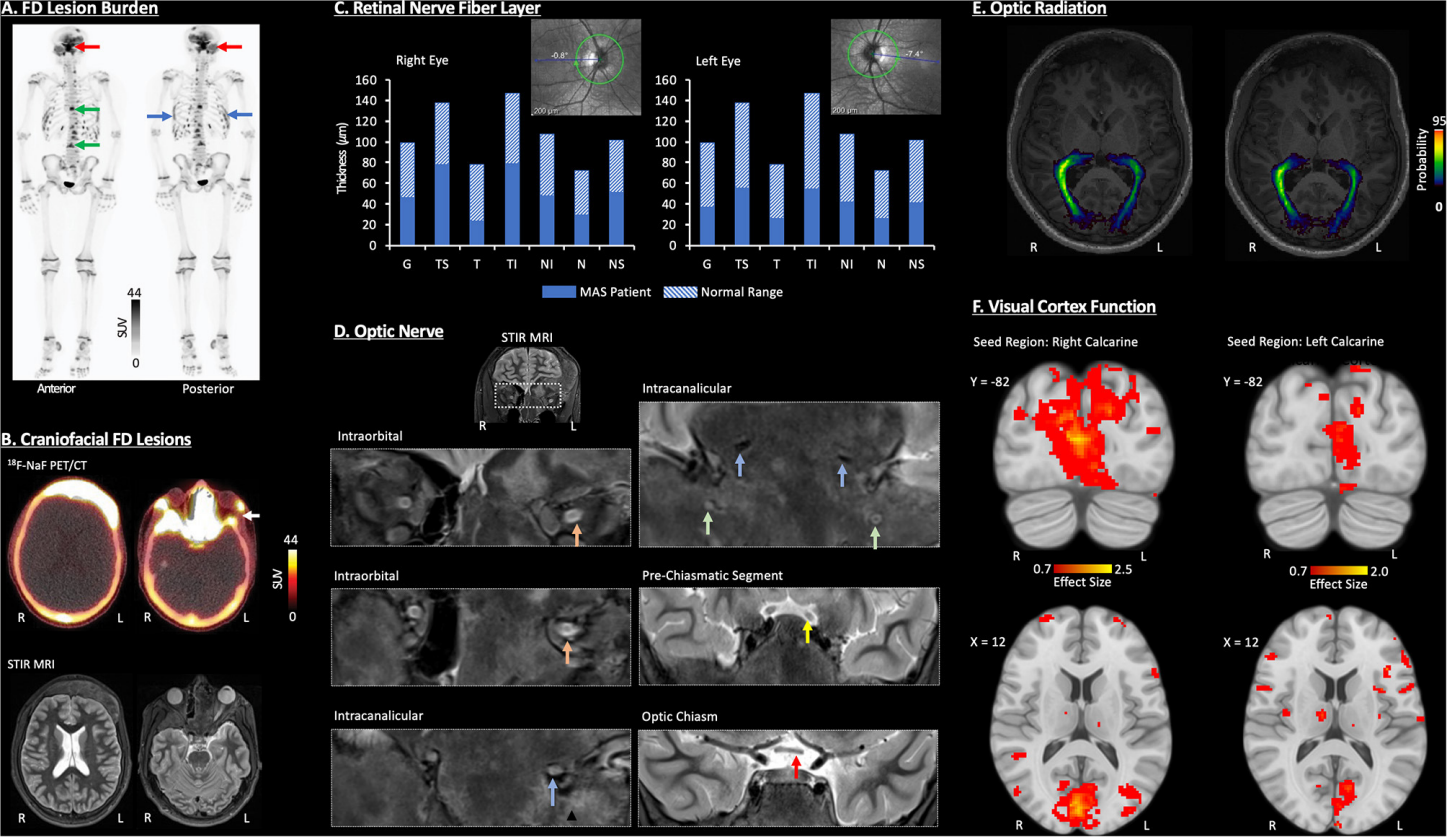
Visual pathway characterization in a pediatric MAS patient. All imaging data were acquired without sedation and at 3-months post-decompression surgery of the left optic nerve. (A) Whole-Body ^18^F-Sodium fluoride positron emission tomography/computerized tomography (^18^F-NaF PET/CT) identified FD lesions within craniofacial bones (red arrow), spine (green arrow), and ribs (blue arrow). (B) A combination of ^18^F-NaF PET/CT and non-contrast MRI identified FD in multiple craniofacial regions (i.e., frontal bones, nasal turbinates, sphenoid bone, greater wing of the sphenoid, zygomatic arch, mastoid, mandible occipital bone, and right maxillary sinus). The white arrow denotes the segment of bone tissue removed during decompression surgery of the left optic nerve. (C) Retinal nerve fiber layer (RNFL) loss was evident in both eyes as revealed by OCT, with slightly greater abnormalities in the left eye. G, Global Average; TS, Temporal-Superior; T, Temporal; TI, Temporal-Inferior; NI, Nasal-Inferior; N, Nasal; NS, Nasal-Superior. (D) Coronal, non-contrast short-tau inversion-recovery (STIR) MRI showed neuropathy in the left optic and trigeminal nerves. Inferior displacement and flattening were detected within intraorbital (orange arrow) and intracanalicular (blue arrow) segments of the left optic nerve. Bilateral trigeminal nerve (V2) inferior displacement, compression, and atrophy was also present (green arrow). At the pre-chiasmatic level (yellow arrow), inferior displacement, mild atrophy, and subtle hyperintensity indicate mild edema or gliosis of the left optic nerve. Left optic nerve atrophy was present in the optic chiasm (red arrow). (E) DTI revealed a loss of white matter fiber density, as defined by the probability distribution, of the left optic radiation relative to the right. The optic radiation is defined as the axonal bundle projecting between the lateral geniculate nucleus and primary visual cortex. (F) Resting-state functional connectivity was reduced for the left primary visual cortex compared to the right. Further details on all data acquisition and analysis procedures as well as additional study findings have been provided in Supplemental Information.
